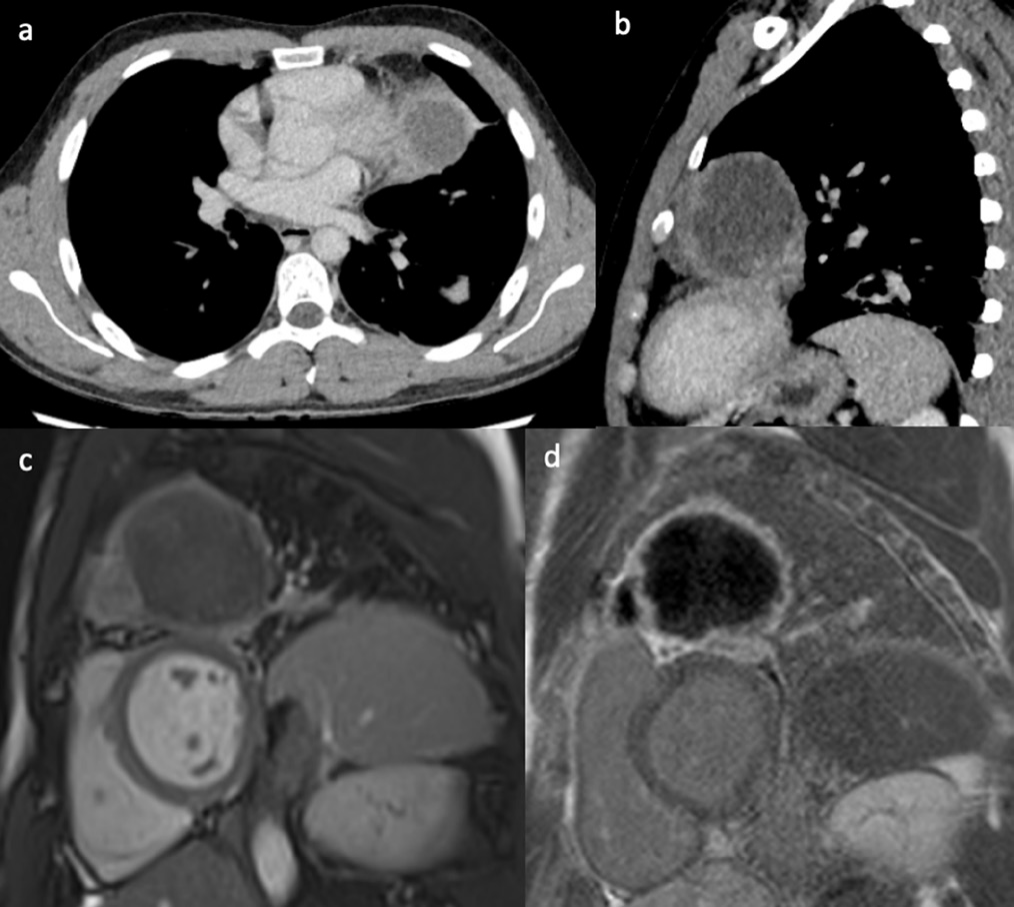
(a, b) Contrast-enhanced CT showing a large left upper lobe lung mass
